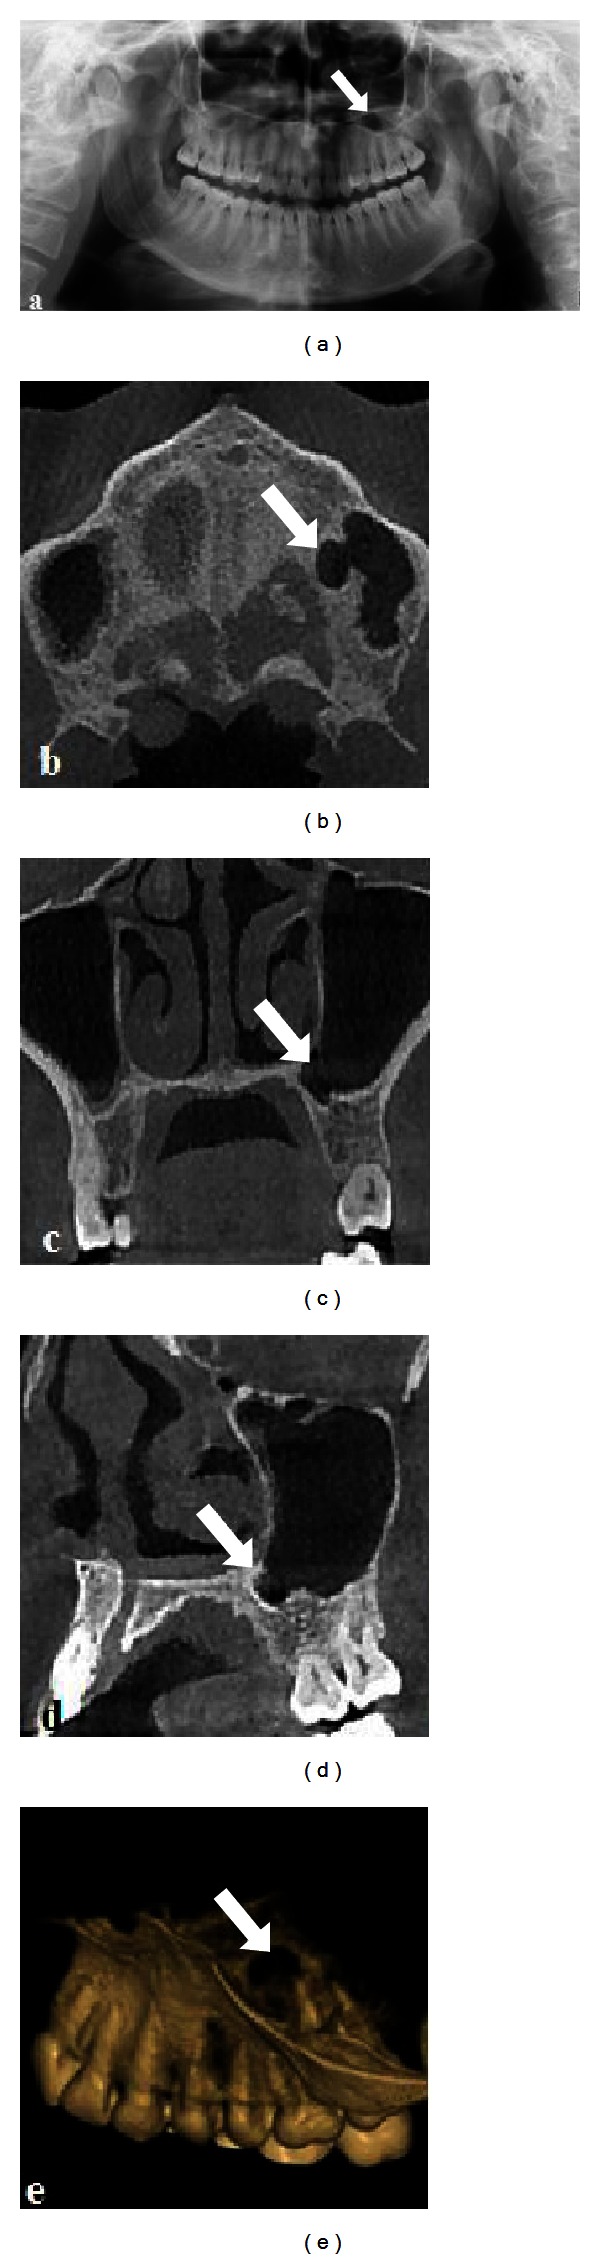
Panoramic radiograph showing well-defined periapical radiolucency in relation to apices of the maxillary left second premolar and first molar (a). Axial (b), coronal (c), sagittal, and three dimensional view of the anatomical variation of maxillary sinus.
